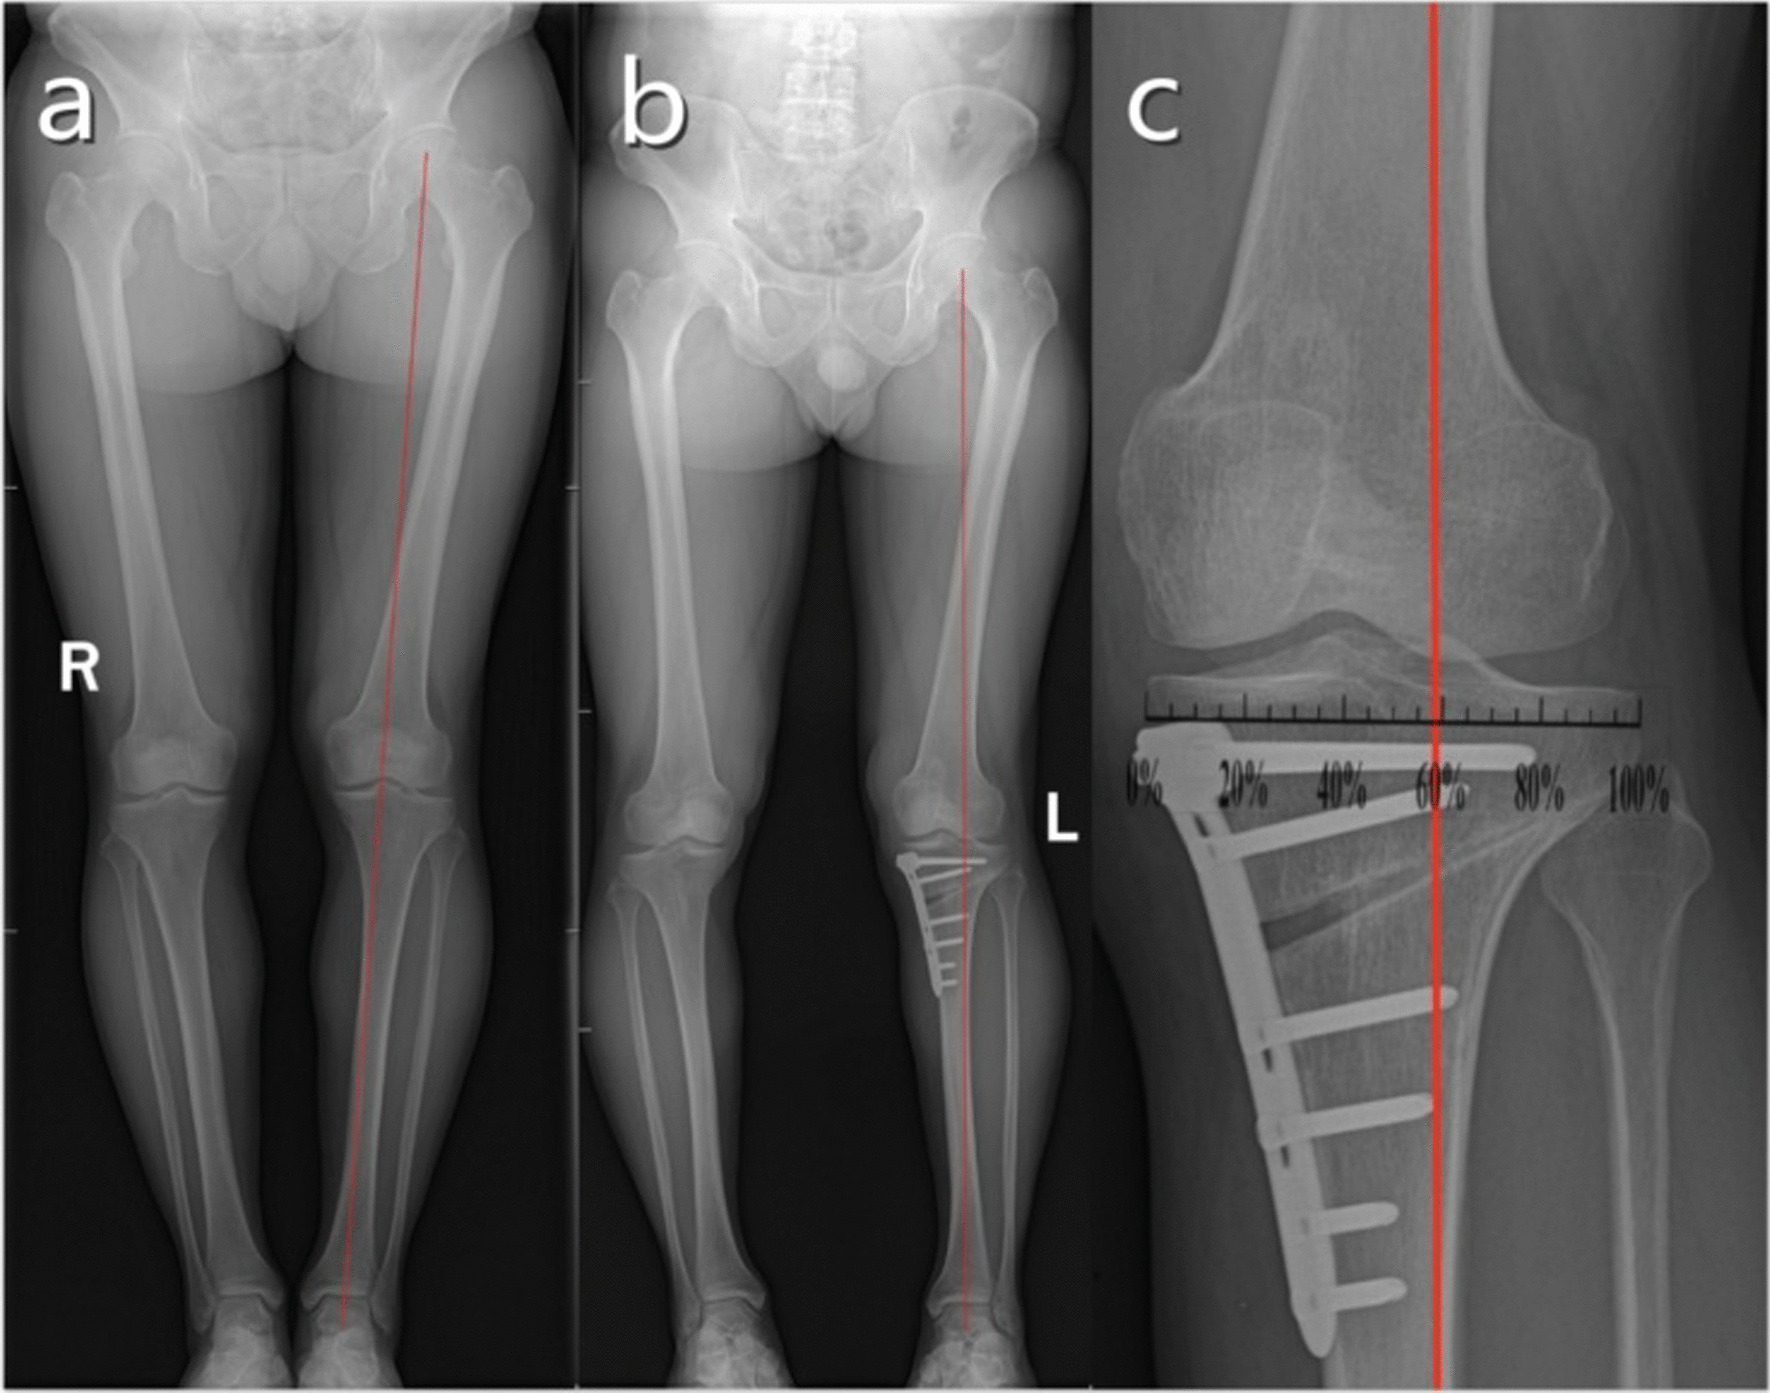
A 52-year-old male was admitted to the hospital for pain in his left knee joint for 6 months and diagnosed with knee osteoarthritis (Kellgren–Lawrence grade 3). Preoperative full-length X-rays of both lower limbs showed that the WBA of the left lower limb deviated from the medial tibial plateau (a). Postoperative X-ray of HTO showed that the WBA of the left lower limb deviated to the lateral tibial plateau (b). After correction, the WBA was located at ~ 50%–60% of the tibial plateau (c)
